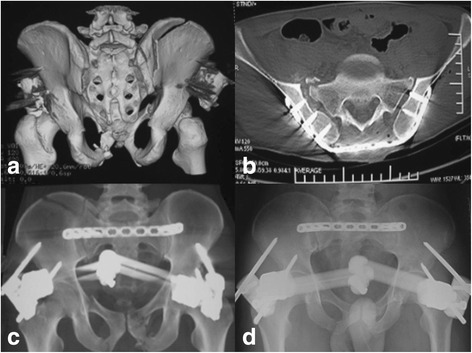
A 48-year-old male patient with anterior and posterior pelvic ring fracture due to a crushing injury. a 3-D CT image showing the anterior pelvic ring fracture. b, c Postoperative X-ray film showing the satisfactory reduction with anterior pelvic external fixation (APEF) and posterior pelvic fixation using locking compression plate (LCP). d X-ray film showing the healed fracture at postoperative 5 months
